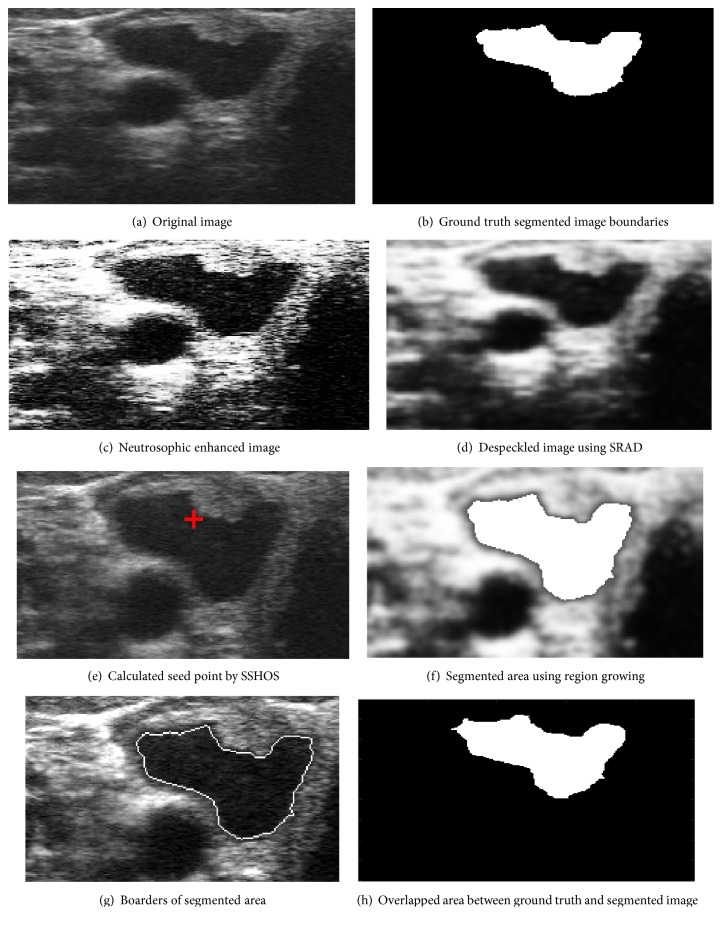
Ultrasound image (image 22B).
